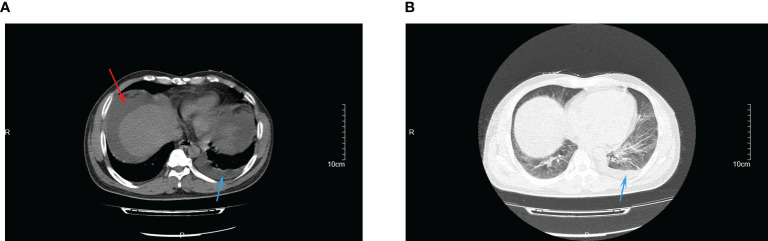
The CT images of abdominal infection (A) and lung infection (B) at admission. Red arrow indicated for ascites; blue arrow indicated for pleural effusion.
